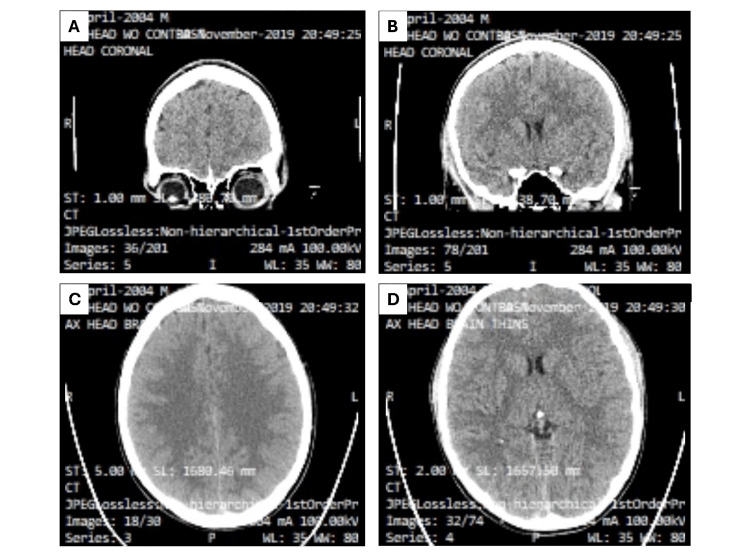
Computed tomography (CT or CAT) scan images. (A-D) Representative computed tomography (CT or CAT) scan images. No acute intracranial pathology was identified as there was no evidence of acute intracranial hemorrhage, midline shift, mass effect or extra-axial fluid collection. The skull base and calvarium were intact. The official radiology interpretation found no clinically significant abnormalities related to the patient's presentation.
